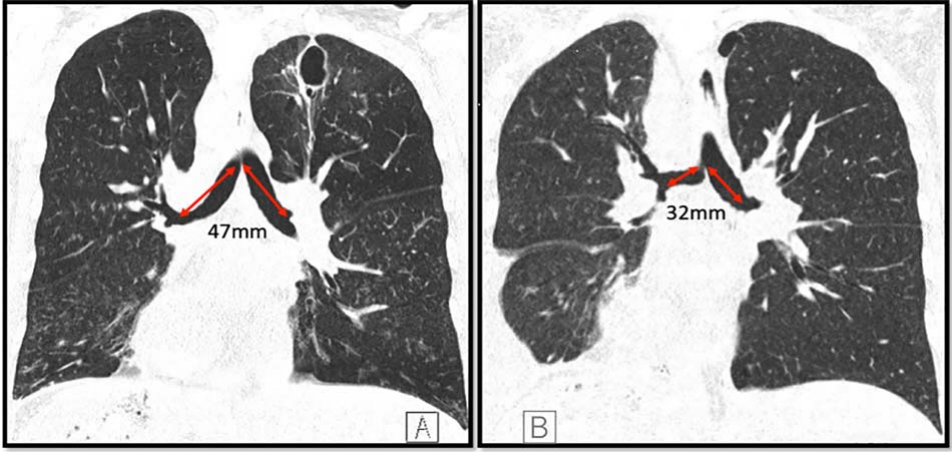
Coronal CT images depicting both main bronchi with the length of 47 mm prior to the lung transplantation (A); length of both main bronchi post transplantation is ~32 mm; the right main bronchus is now at an approximate right angle with the trachea (B); note the presence of dextrocardia in both images and a thin-walled postinfectious left upper lobe cavity in the native lung.
